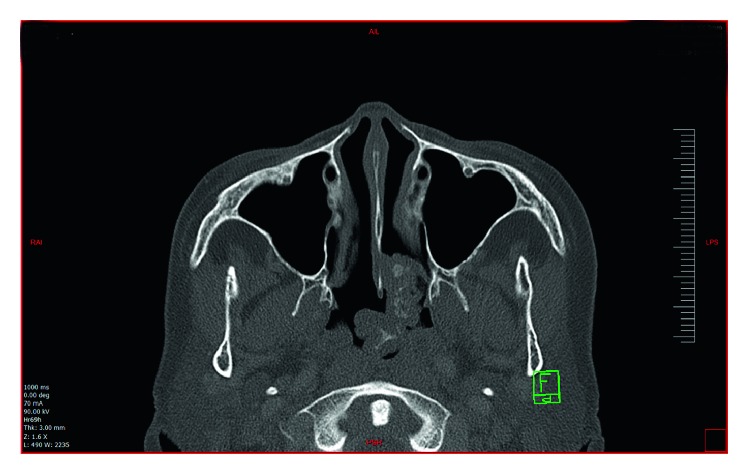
Posterior nasal mass with calcified core. CT performed 1 year following the previous scan (Figure 1).
